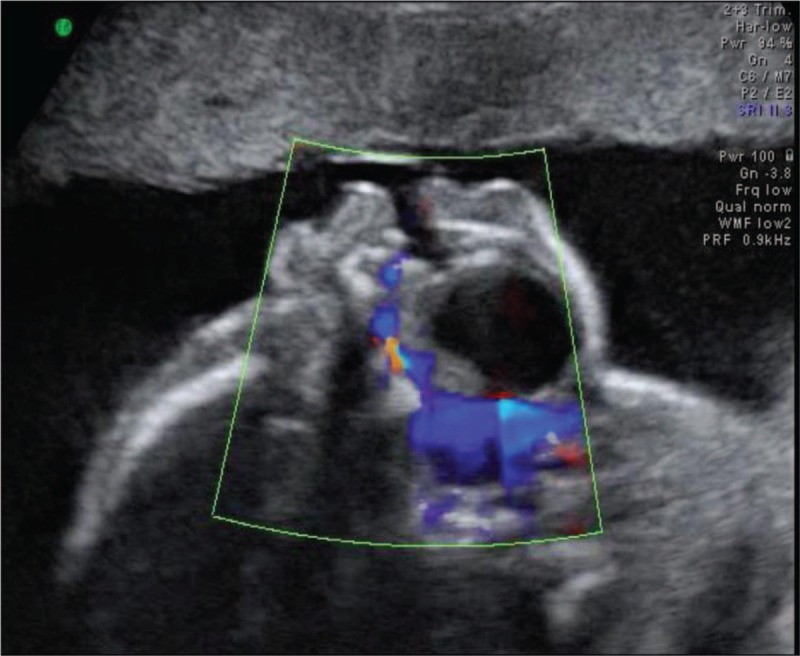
Color Doppler imaging shows the patency of the nasal/nasopharynx, hypopharynx, and trachea.
